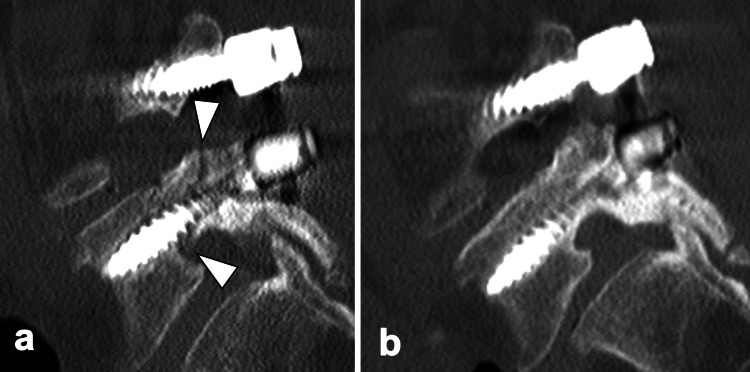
Sagittal computed tomography of the lumbar spine in Case 1 a. Sagittal computed tomography (CT) image obtained five days postoperatively demonstrating bilateral L5 pedicle fractures. b. Sagittal CT image at three years postoperatively demonstrating complete bone union of the L5 pedicle fractures.
